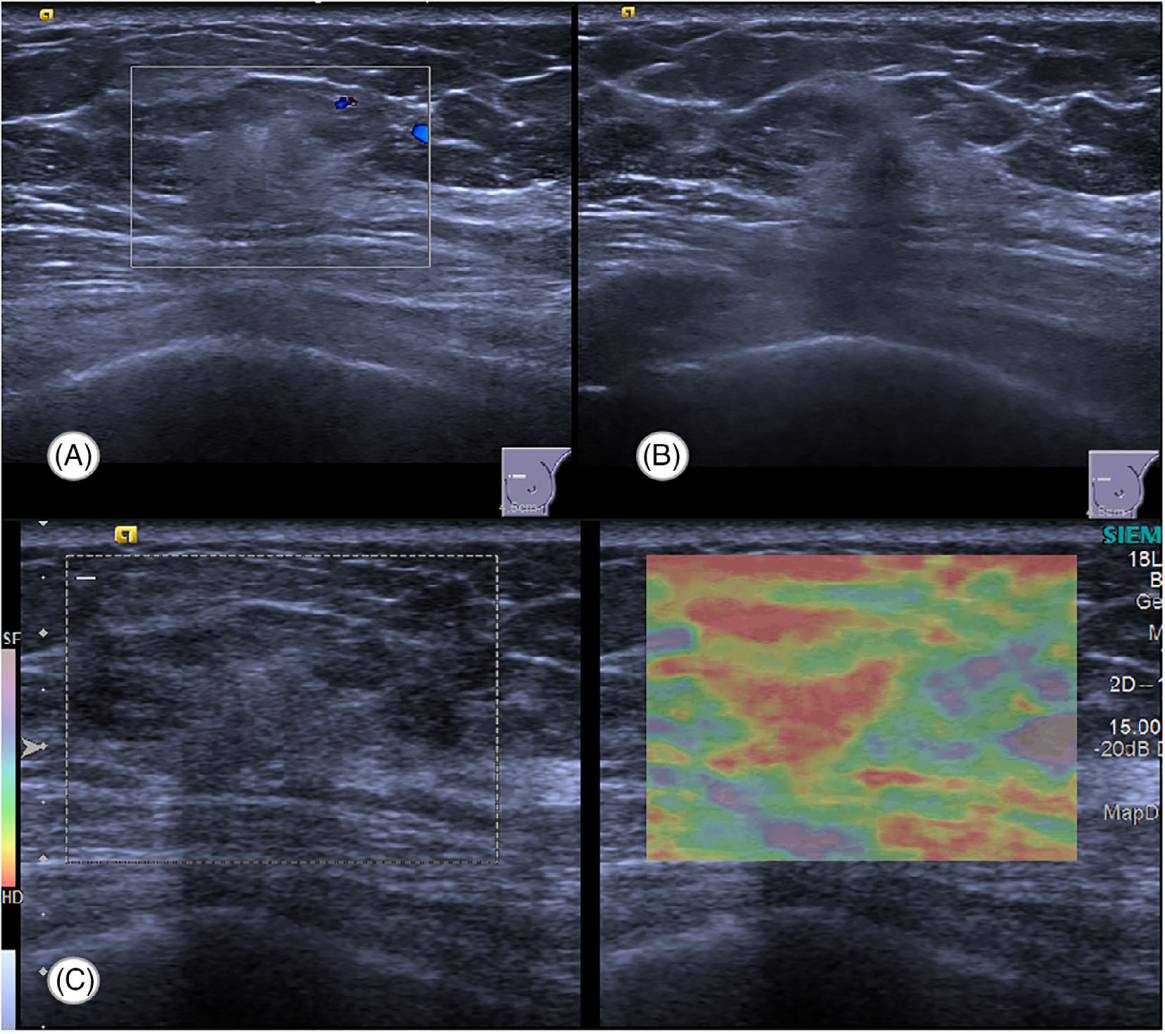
Ultrasonic finding of the left lesion by hand‐held ultrasound (HHUS). (A) Color Doppler sonogram shows no internal vascularity within the lesion. (B) Gray‐scale transverse view of the left breast demonstrates a heterogeneous hypoechoic lesion in the 10 o'clock position. (C) Elastography revealed a Tsukuba stiffness score of 3
